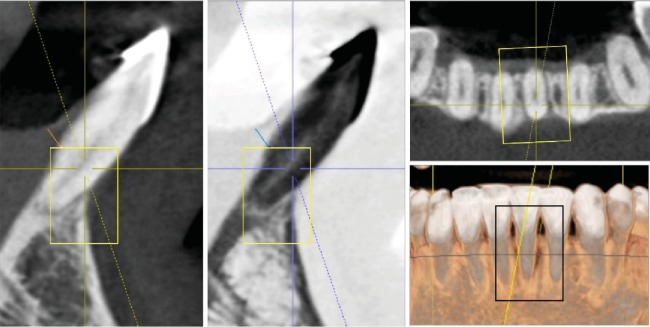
Final CBCT of the left mandibular central incisor. Sagittal, coronal, and axial views.
